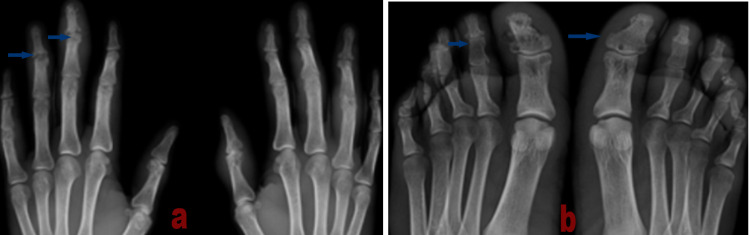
Radiograph of the hands and feet. (a) Radiograph of the hands showing multiple geodes and osteolysis at the proximal and distal interphalangeal joints. (b) X-ray of the feet showing subchondral geodes facing the interphalangeal space of the bilateral hallux and osteolysis of the fourth right ray.
